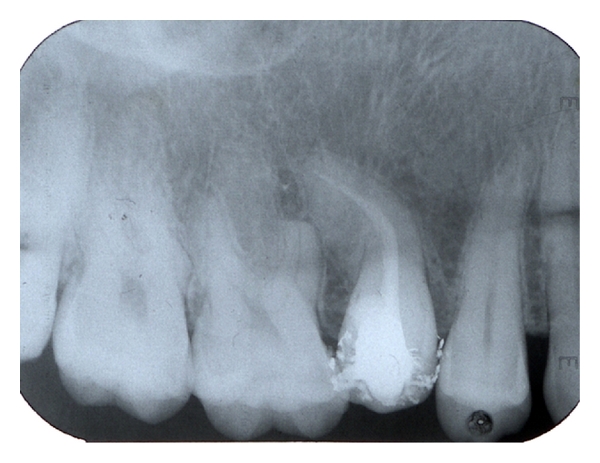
Postoperative radiograph following root canal treatment of the premolar.
